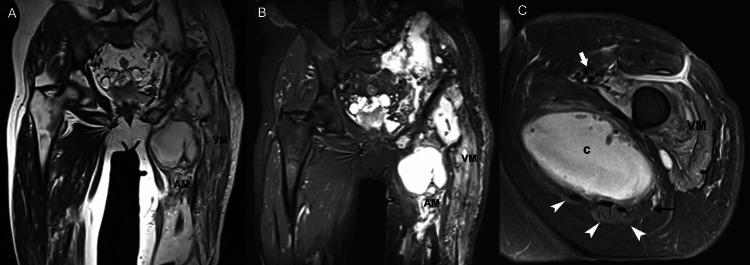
A 65-year-old man with generalized bone and soft-tissue echinococcosis of the left hemipelvis and the thigh. Coronal T2-weighted (A) and short-tau inversion recovery (B) images show diffuse edema in the vastus intermedius and lateralis muscles (VM) coupled with marked atrophy of the adductor (AM) muscles, in contrast with the normal appearance of the opposite side. (C) Axial short-tau inversion recovery image through the thigh shows the denervation of the vastus muscles (VM) owing to the inflammation of the femoral nerve (thick arrow). A large parasitic cyst (c) growing within the adductor magnus muscle causes compression of the sciatic nerve (arrow), and atrophy of muscles in the posterior compartment of the thigh (arrowheads).
